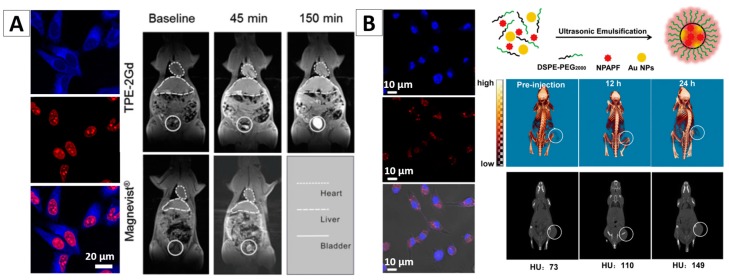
(A) Cell imaging and in vivo MIR imaging with TPE-2Gd NPs [78]; (B) Molecular structures of co-encapsulation of NPAPF and Au by DSPE-PEG2000 and their application in fluorescent cell imaging and in vivo CT imaging [65]. Reprinted with permission from [65,78], respectively. Copyright 2016 Elsevier and Copyright 2014 American Chemical Society, respectively.
